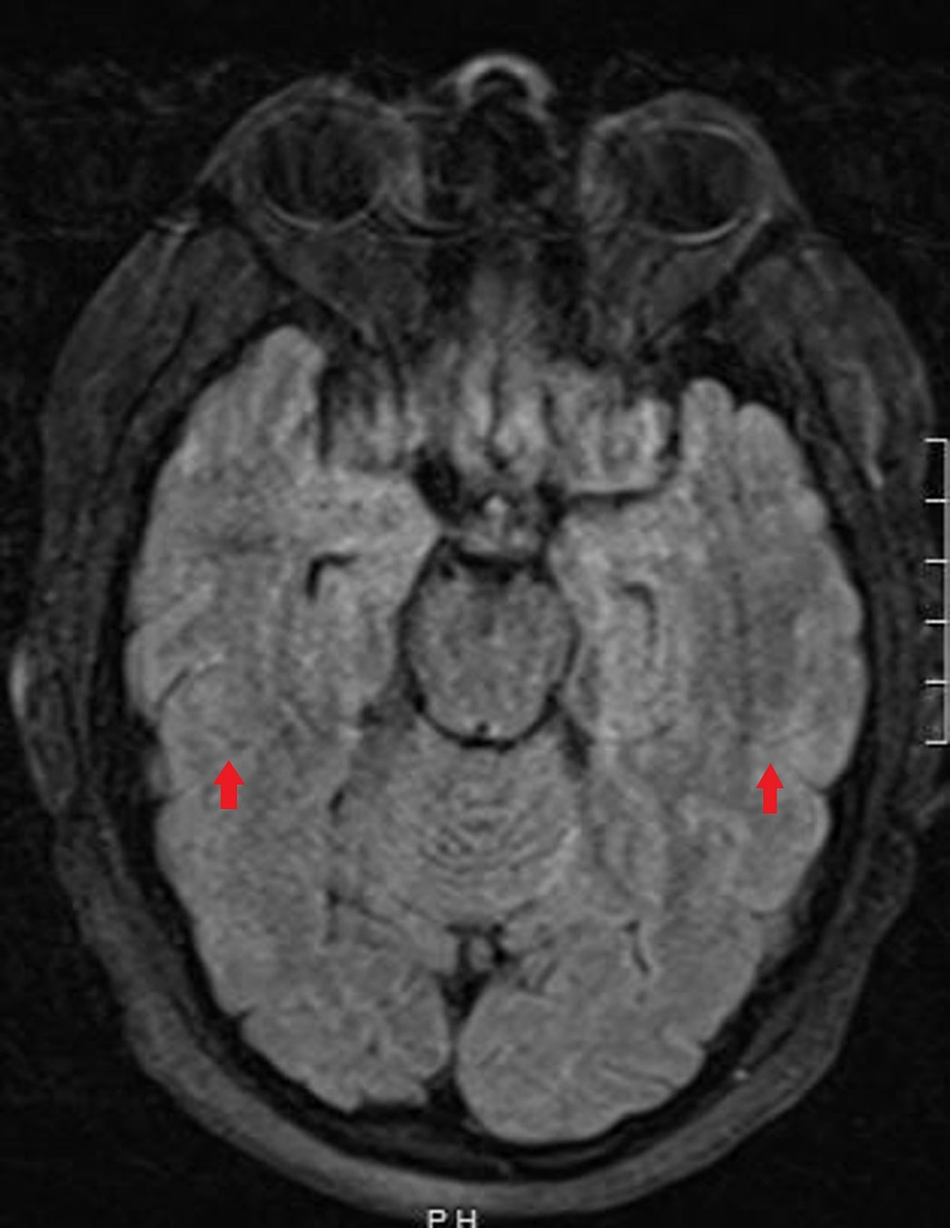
Magnetic resonance imaging (MRI) of the brain Normal brain MRI with no abnormal leptomeningeal or intraparenchymal enhancement (red arrows). No hemorrhage, mass, or acute infarct seen.
